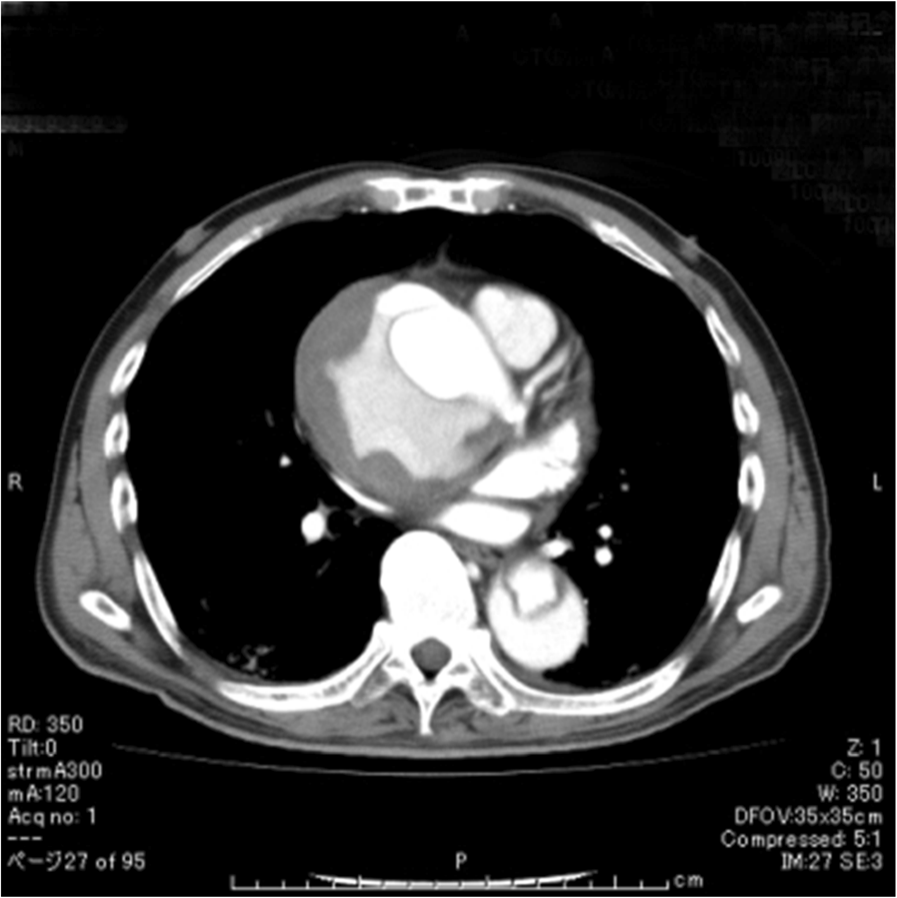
CTA showing enlargement of the ascending aortic width to 80-mm.
